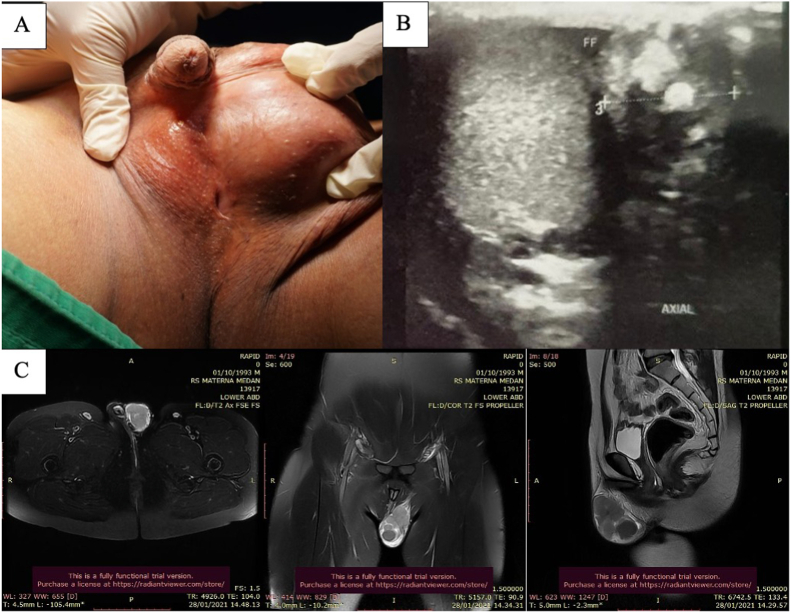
Preoperative evaluation A. Clinical Picture of the Left Paratesticular Tumor and Scrotal Hypospadia B. Perioperative Testicular USG C. MRI of patient with paratesticular seminoma.
